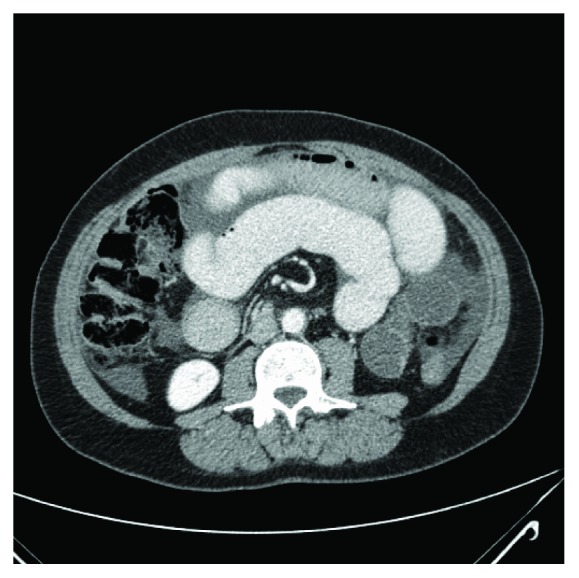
CT of the abdomen. Whirl sign at the base of the mesentery around the superior mesenteric artery consistent with midgut volvulus.
